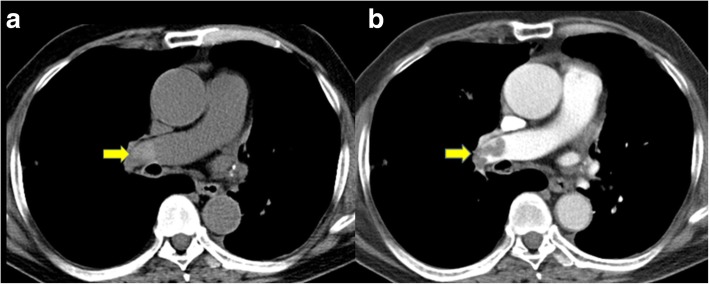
This patient was seen in the emergency department with dyspnea and diagnosed with an acute pulmonary embolism by CTPA. a Non-contrast computed tomography showed high attenuation emboli in the right pulmonary artery (arrow). b Post-contrast image showed filling defects in the right pulmonary artery
